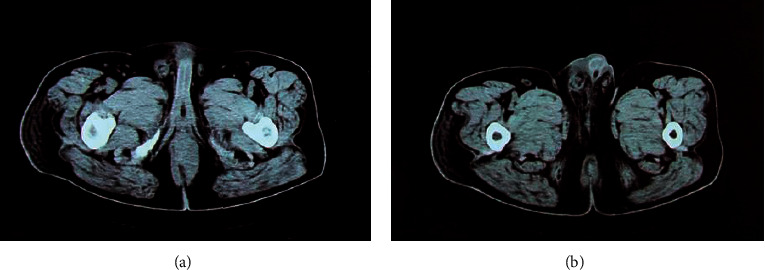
CT image with the spread of abscess upward to the left levator ani muscle, forming an abscess (case 2, a male patient, aged 56 years). (a) Axial CT image. (b) Coronal CT image.
